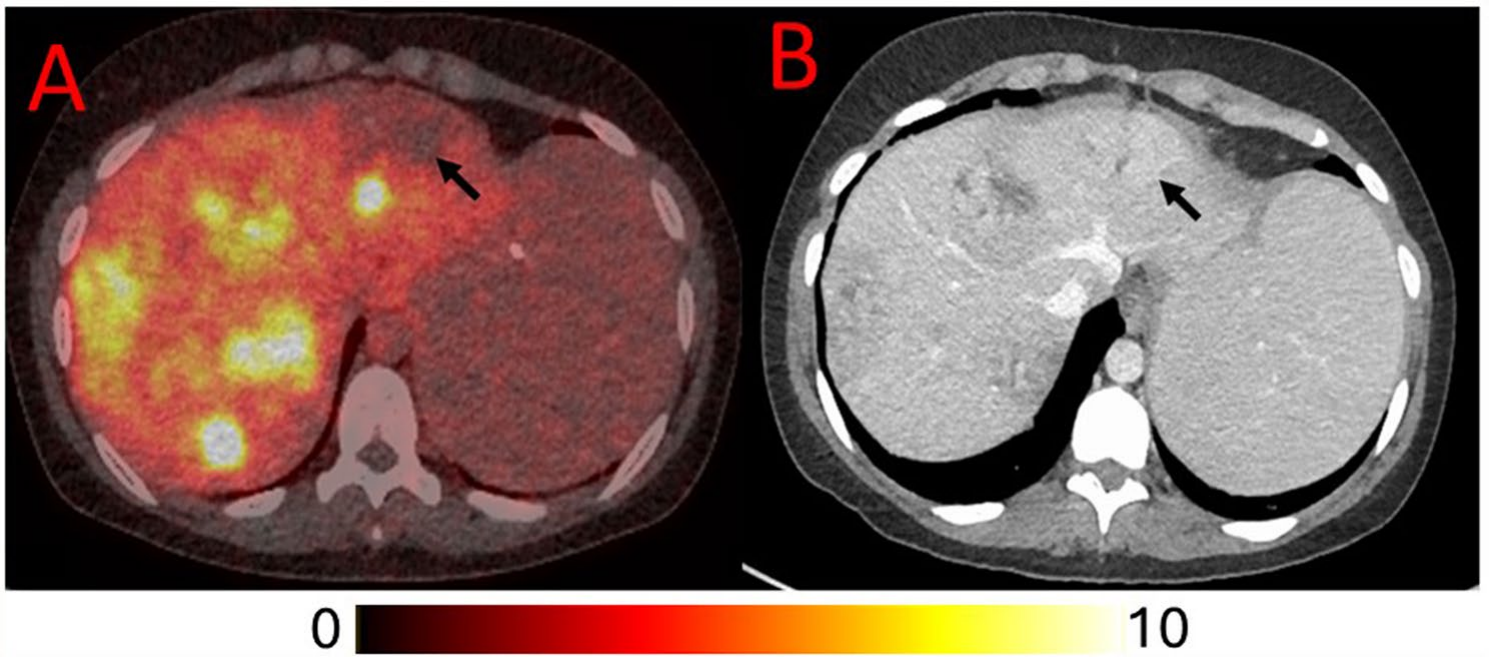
(A) Axial fused [^68^Ga]NeoB PET/CT demonstrating heterogenous uptake within liver metastases and (B) Correlative staging CT Study showing the extent of liver disease. Multiple enhancing lesions in the right lobe demonstrate NeoB uptake, however a further enhancing lesion within the left lateral section (arrow) corresponds to a region on photopaenia on the PET images
